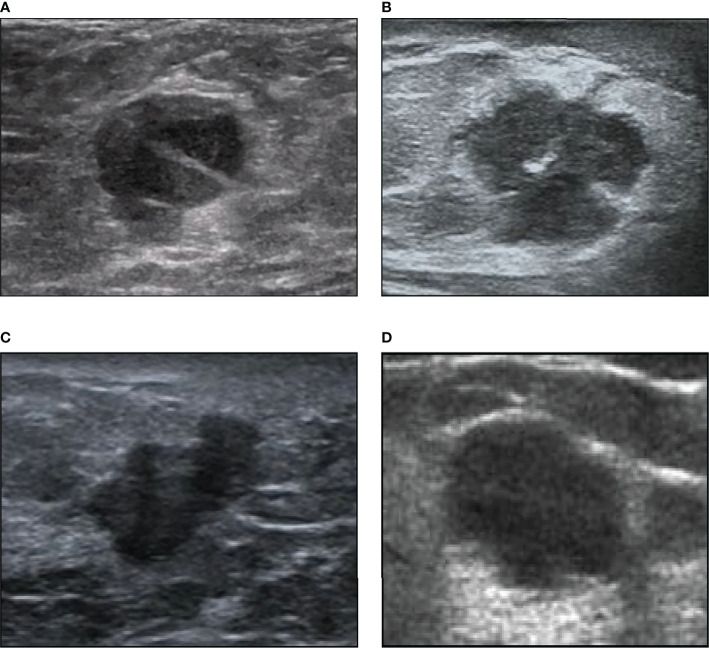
Examples of our ultrasound image dataset. (A) A 72-year-old woman with intraductal papillary breast carcinoma (1.6 cm) of luminal A subtype. (B) A 56-year-old woman with invasive ductal breast carcinoma (1.7 cm) of luminal B subtype. (C) A 40-year-old woman with invasive micropapillary breast carcinoma (1.5 cm) of HER2+ subtype. (D) A 39-year-old woman with invasive ductal breast carcinoma (1.9 cm) of triple-negative subtype.
